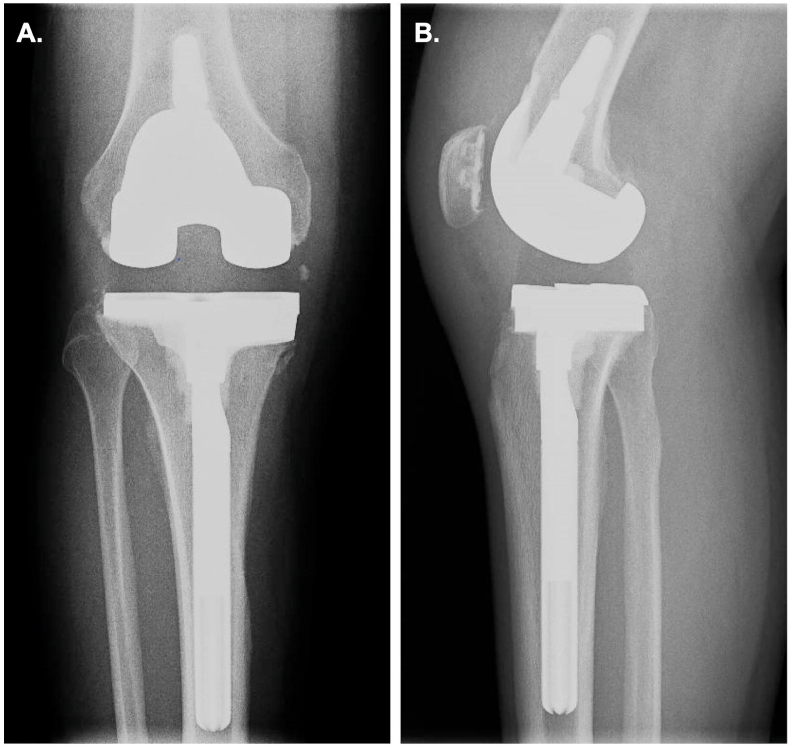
Anterior (A.) and lateral (B.) weight bearing X-ray films of the right knee on day +621 after revision of Zimmer Persona total knee arthroplasty (TKA) was performed on day +561.
